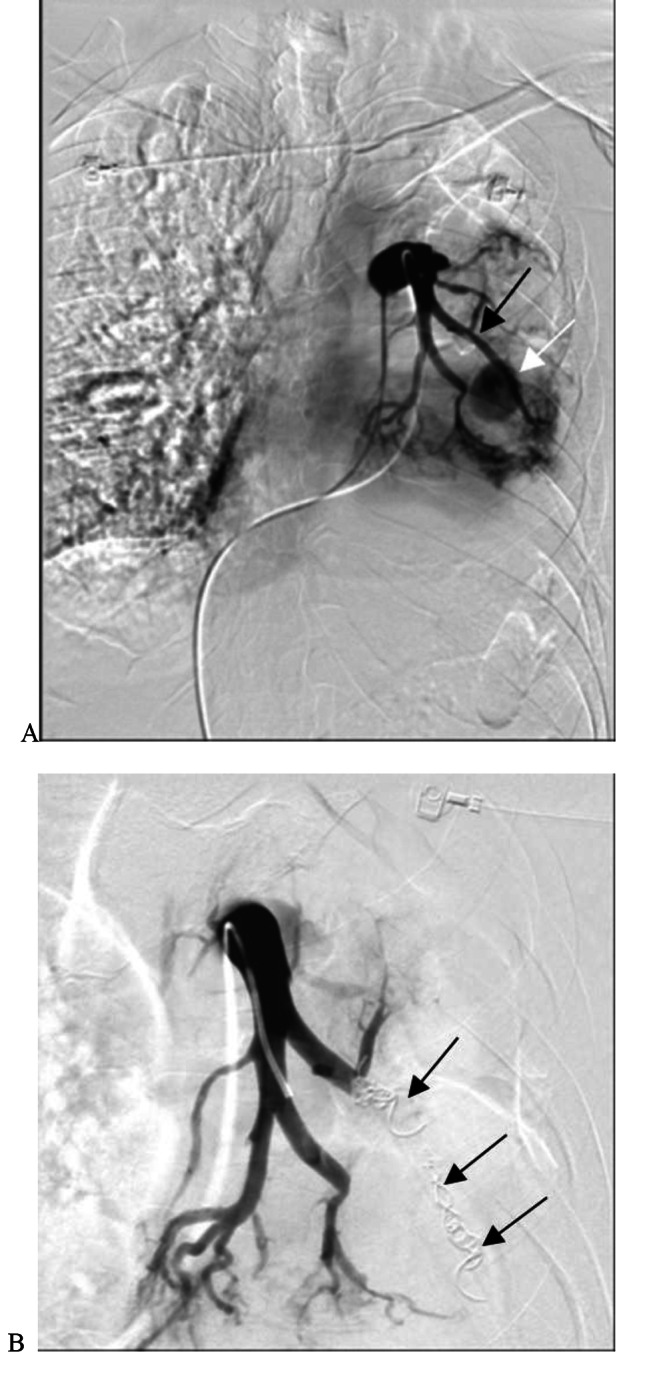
Sudden massive hemoptysis (about 600ml) of unknown cause. Contrast-enhanced CT showed a pseudoaneurysm of the left inferior pulmonary artery. A. Left pulmonary artery angiography shows the left inferior pulmonary artery (black arrow) was PAP (white arrow). B. The distal pulmonary artery (white arrow) of the left lower lobe of the lung was superselected with a microcatheter. Complete embolization was performed from the distal end to the proximal end with interlock coils (black arrow)
